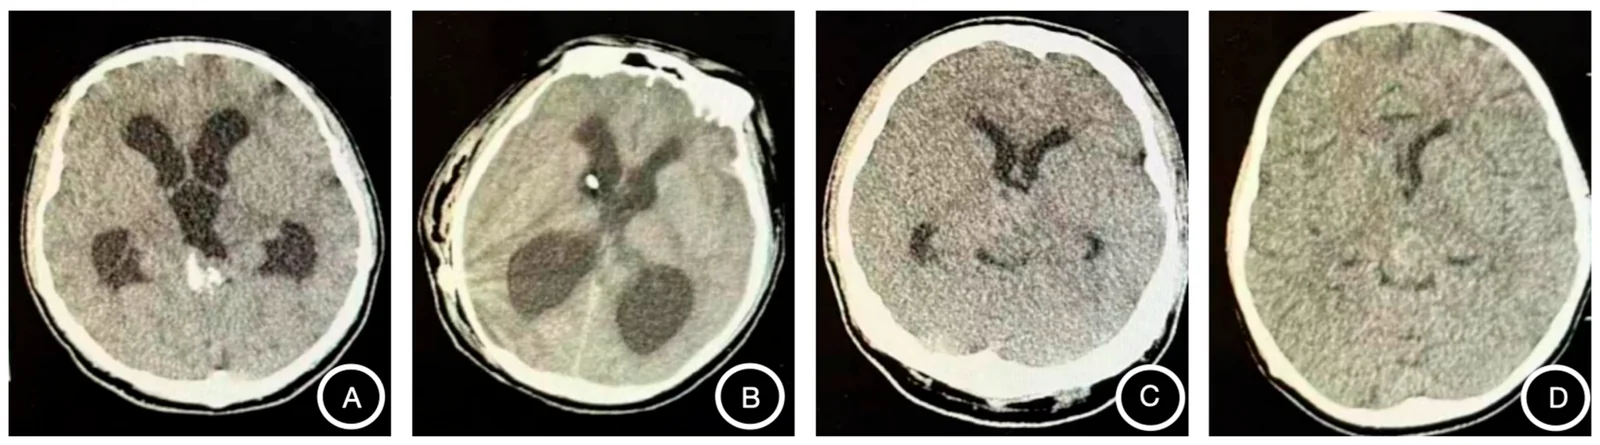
Axial computed tomography images. (A) Case 1, a 17-year-old male: irregular mixed-density mass in the posterior third ventricle/pineal region with multiple calcifications. (B) Case 2, a 15-year-old male: posterior third ventricular isodense mass with punctate and plaque-like calcifications. (C) Case 3, a 19-year-old male: irregular mildly hyperdense pineal region mass. (D) Case 4, an 8-year-old male: irregular mildly hyperdense pineal region mass with heterogeneous density and small hypodense foci.
